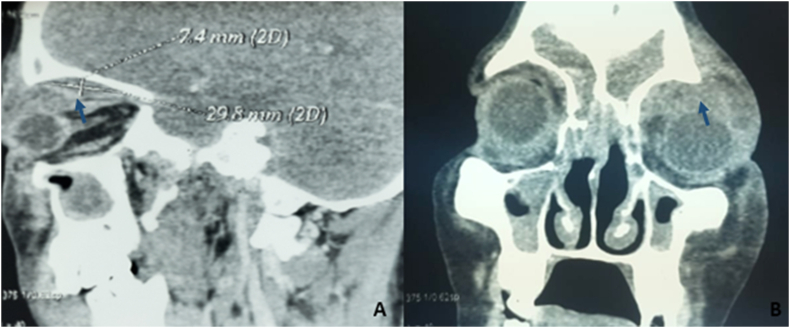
A CT-scan, showing a left orbital cellulitis and a 7.4mm × 29.8mm subperiosteal abscess complicating pansinusitis (blue arrow). A: Sagittal view, B: Frontal view. (For interpretation of the references to colour in this figure legend, the reader is referred to the Web version of this article.)
